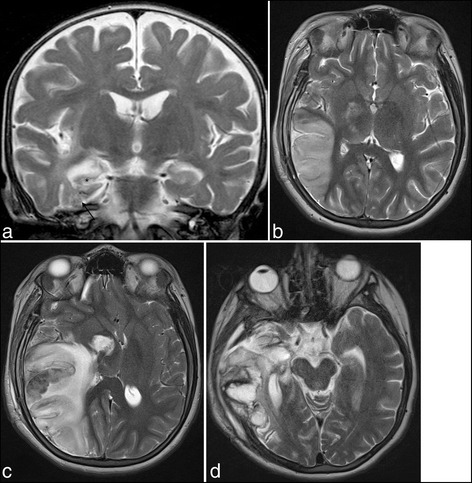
Sequels of HSVE at the level of the temporal lobe and right hippocampus (3a, upper left, arrows and asterisk). Post-surgical MRI shows areas of suspected encephalitis with high signal on T2 in the right frontal, parietal and temporal lobes at 3 weeks (3b, upper right), associated with hemorrhagic transformation and mass effect one month later (3c, lower left). Follow-up MRI at 45 days showed large sequelae of the temporal lobe (3d, lower right)
